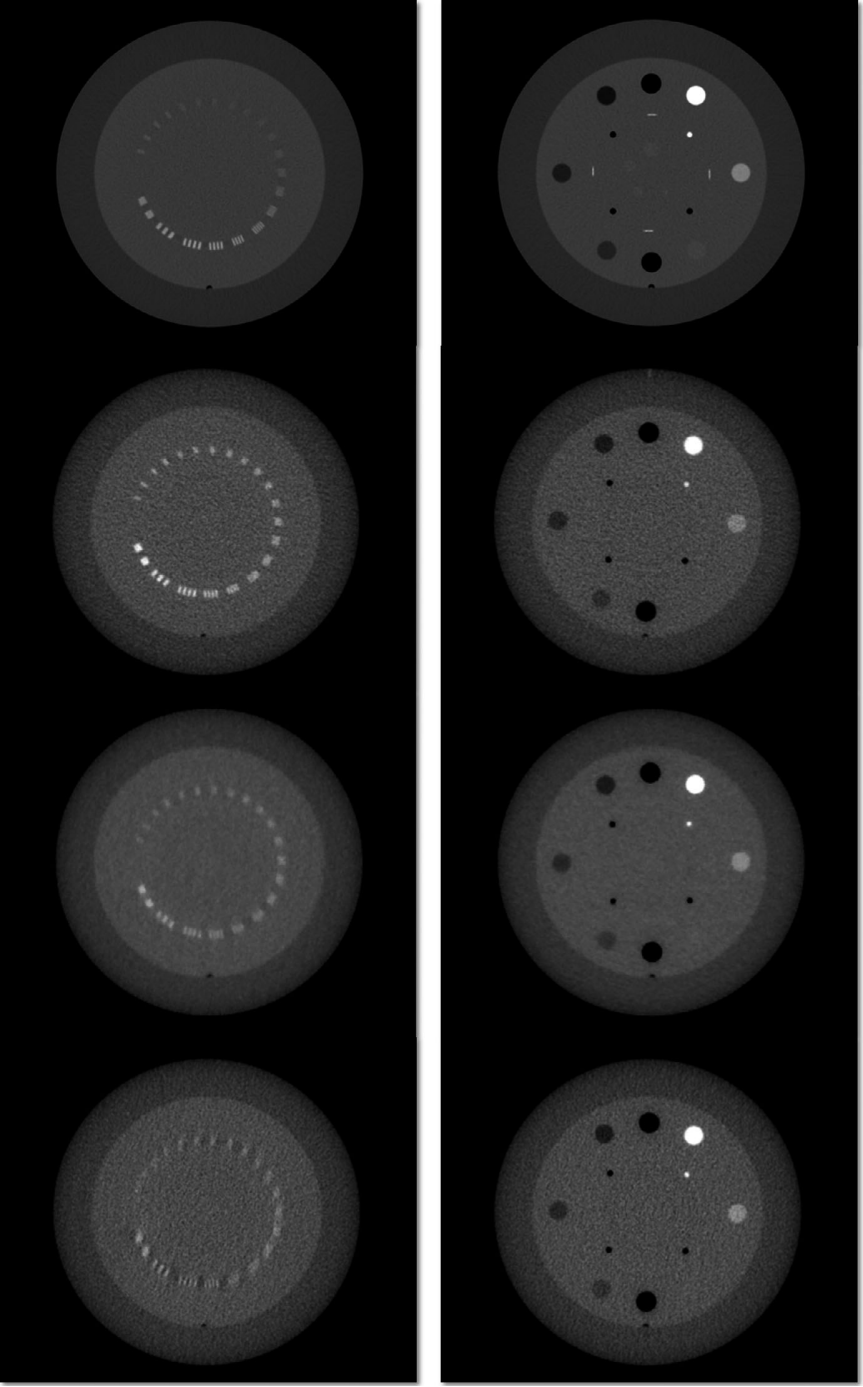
Computed tomography slices for standard protocol kilovoltage CT with 2.5 mm slice thickness (row 1) and fine, normal, and coarse pitch (rows 2–4) with standard reconstruction megavoltage CT with 1, 2, and 3 mm slice thicknesses.
